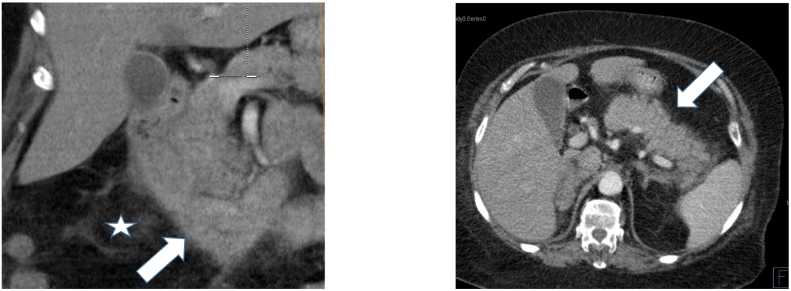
(A): CT coronal reconstruction with contrast injection showing a regular circumferential parietal thickening of the D2, D3 portions of the duodenum (arrowheads), swollen pancreas (arrow) with densification of the mesenteric fat around (star), B: axial reconstruction with contrast injection showing a swollen pancreas (arrow).
